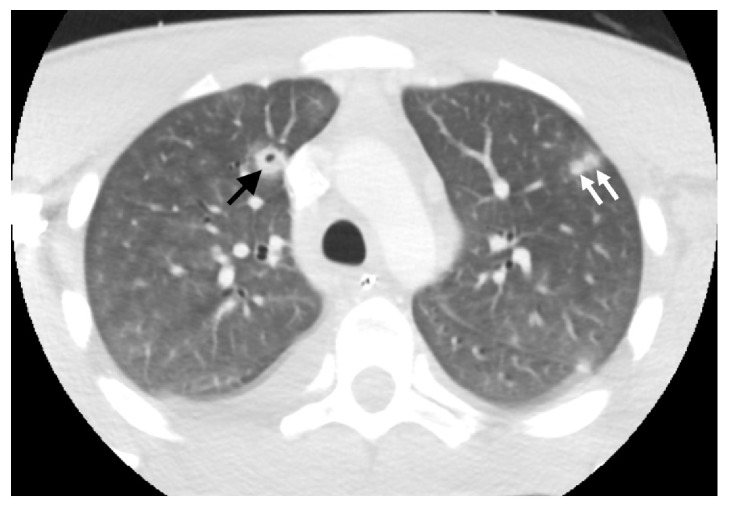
Axial image through the upper chest from CT scan of the neck. Multiple nodules were visualized in the imaged portion of the chest, including cavitary (black arrow) and noncavitary nodules (white arrows).
